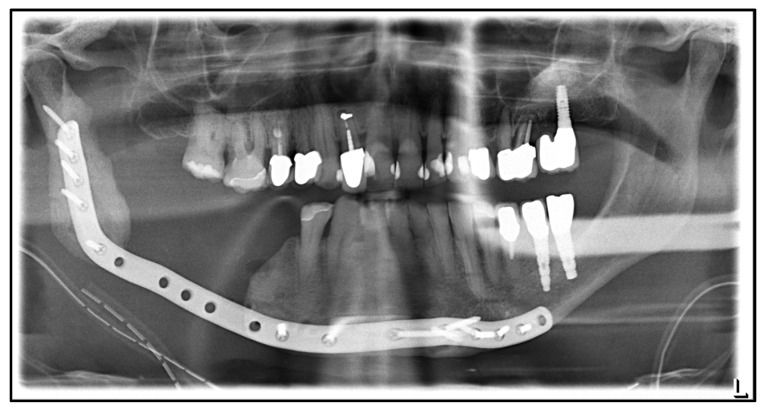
Dental X-ray after mandibular continuity resection and osteosynthesis with a patient-specific plate, August 2018.
